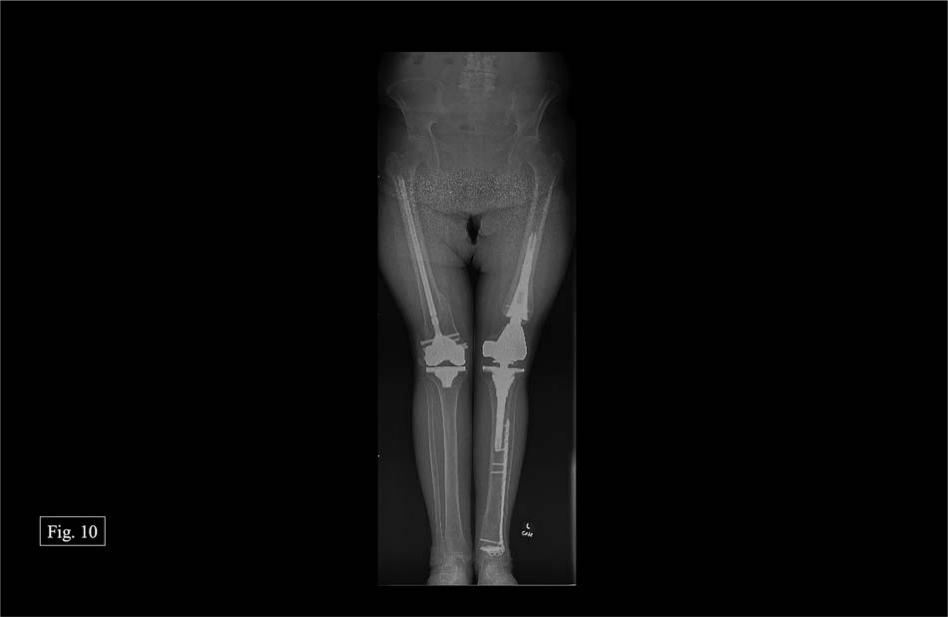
Bilateral, February 2024, full leg length X-ray.
